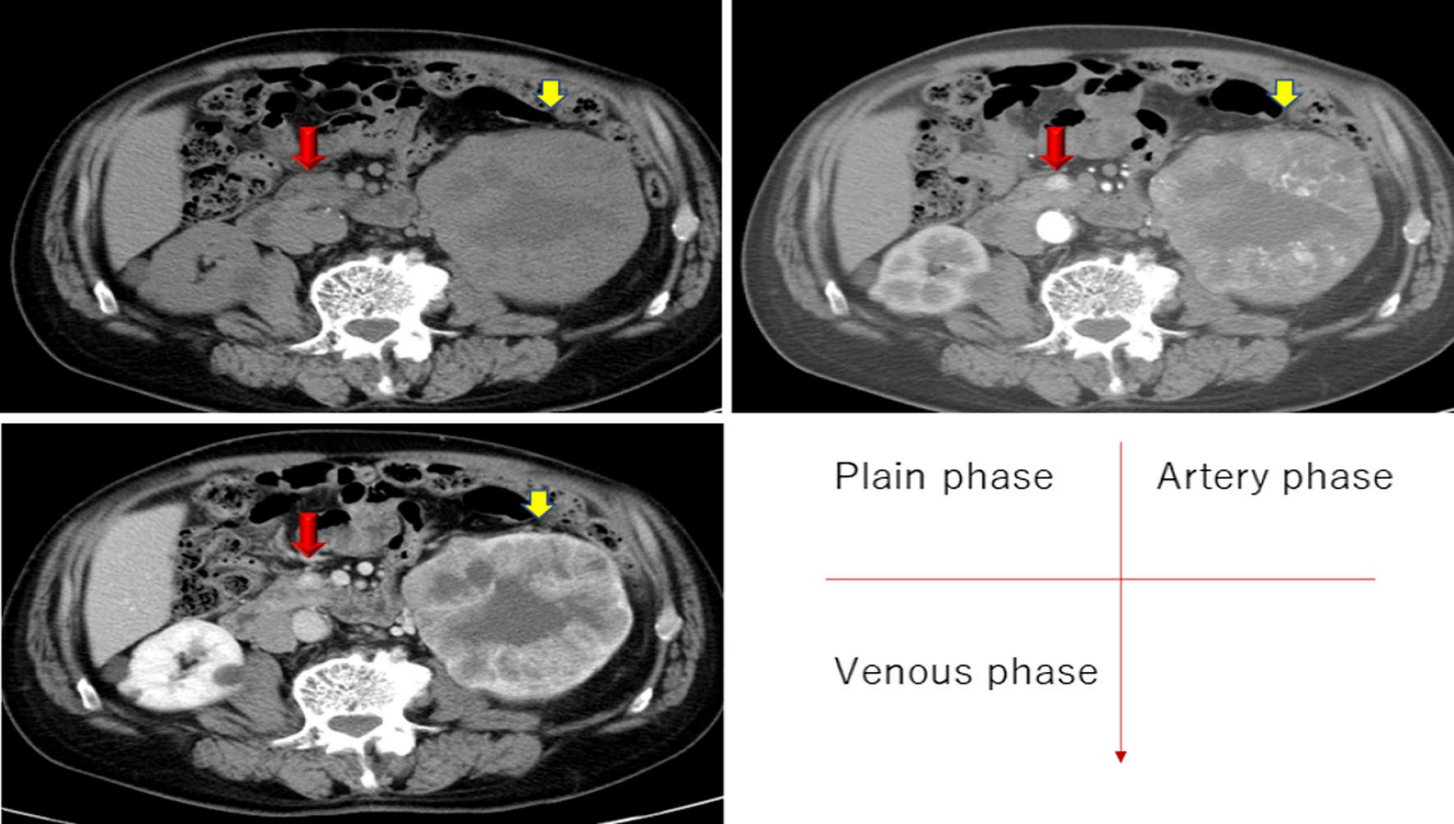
Enhanced CT findings of the RCC and pancreatic head tumor. The renal tumor (yellow arrow) was 10 cm in diameter and demonstrated enhancement in the arterial phase and even more enhancement in the venous phase, but less enhancement than that in the normal right kidney. The tumor was diagnosed as an RCC with necrotic components. The pancreatic head tumor (red arrow), measuring 1 cm in diameter, was well enhanced in both the arterial and venous phases. The tumor was diagnosed as a metastasis from the RCC or a primary PNET
